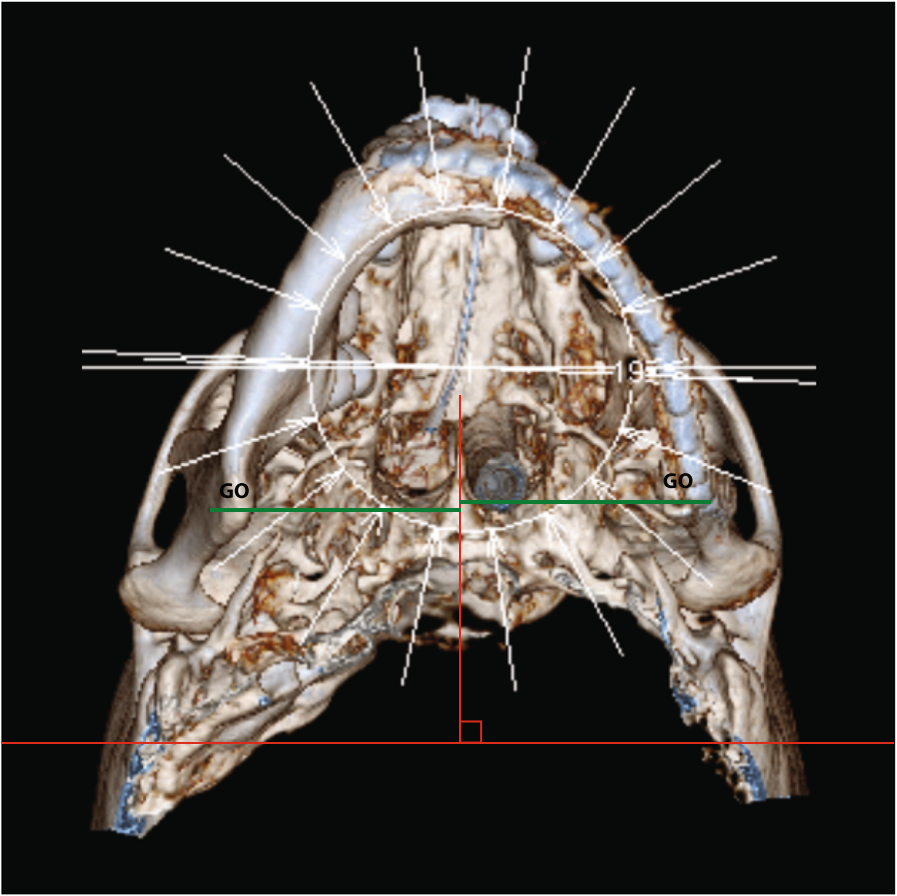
Medial-lateral distances between gonion points (GO) and vomer line (VL) in the 3D-CT image (submentovertex view) were measured and compared in all participants
